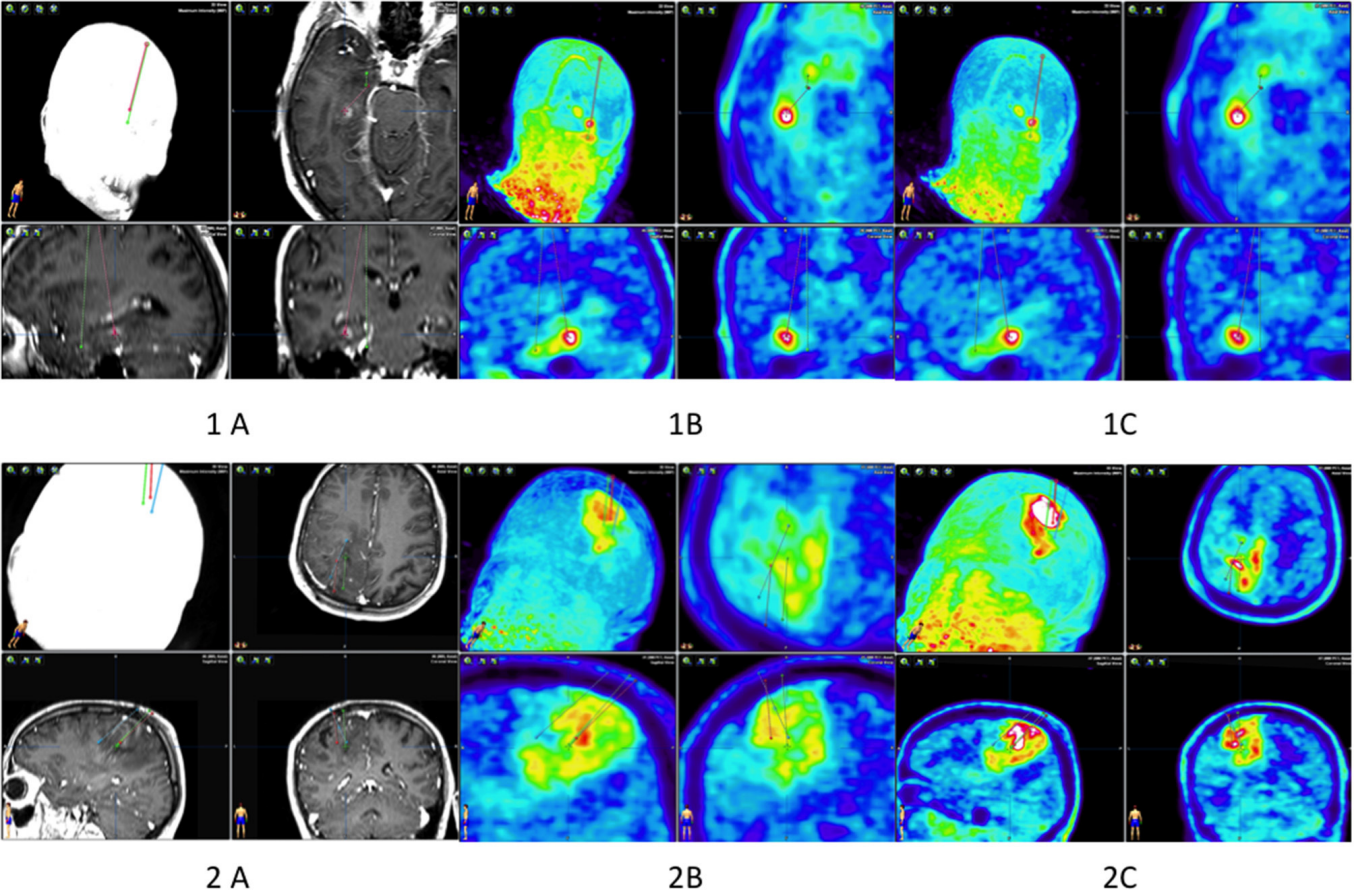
Biopsy planning of patients 1 and 2. Patient 1: two biopsy trajectories in the MRI (A), in PET 10 (B), and in PET 60 (1C). Patient 2: three biopsy trajectories in the MRI (A), in PET 10 (B), and in PET 60 (2C).
